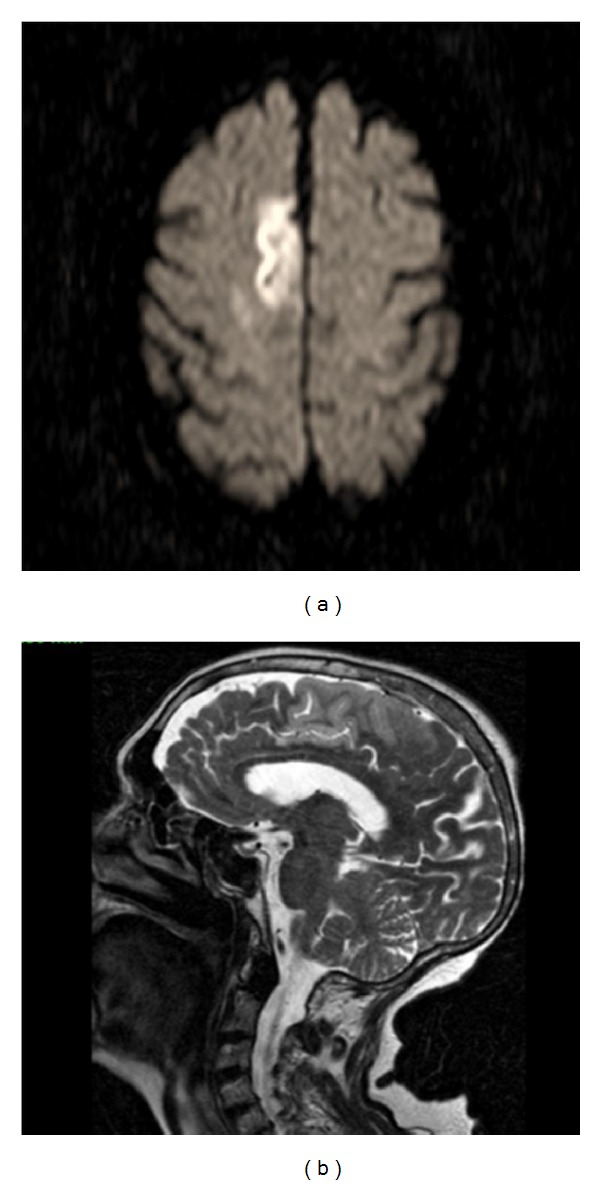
(a) Diffusion-weighted image and (b) T2-weighted image: right cortical and subcortical frontal parasagittal acute ischaemic lesion, affecting the superior frontal gyrus and the cingulate gyrus.
